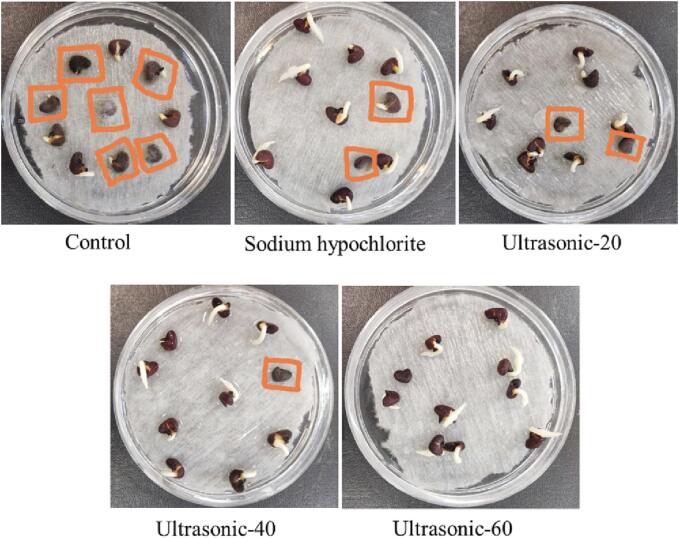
Photographs of control seeds and seeds subjected to sodium hypochlorite 1.5% for 20 min, ultrasound treatment for 20 min, 40 min and 60 min and their effects on seed rot after three days of storage at room temperature. The rotten seeds in each treatment were shown by pink color.
